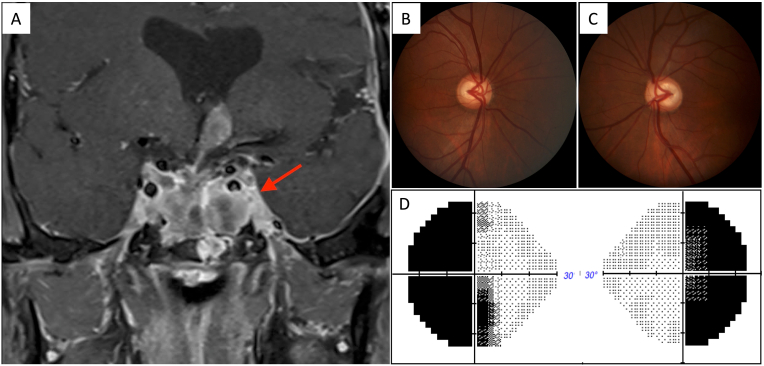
A: Magnetic resonance imaging revealed a heterogeneous mass (red arrow) in the sellar and suprasellar regions, as well as bilateral cavernous sinus. Right (B) and left (C) fundus photographs showed cup-to-disc ratio of 0.6 in the right eye and 0.7 in the left eye with mild pallor nasally and temporally in both eyes. Humphrey 24–2 visual fields (D) showed a bitemporal hemianopia. (For interpretation of the references to colour in this figure legend, the reader is referred to the Web version of this article.)
